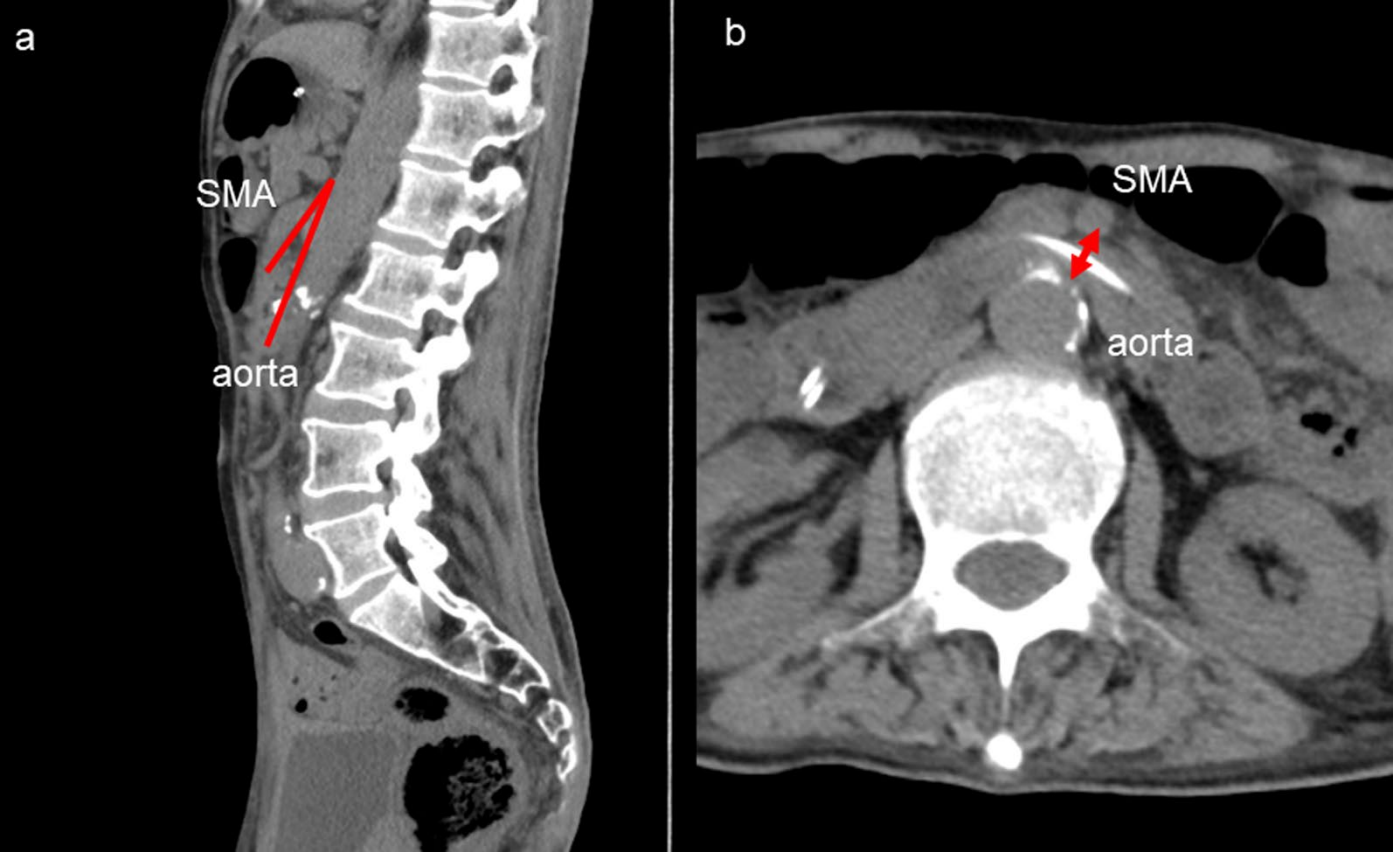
Computed tomography findings. a Sagittal view. b Axial view. The aorto-mesenteric angle is narrow at 18° and the aorto-mesenteric distance short at 8 mm
